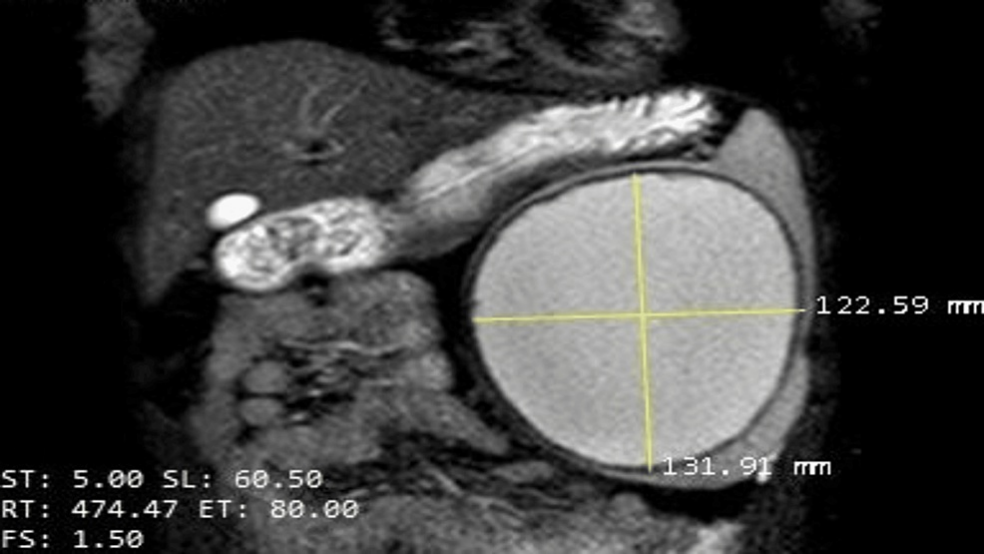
A unilocular cystic mass measuring (13×12 cm) size was seen on T1-, T2-weighted magnetic resonance image (MRI) showing fluid signal intensity within the cyst
